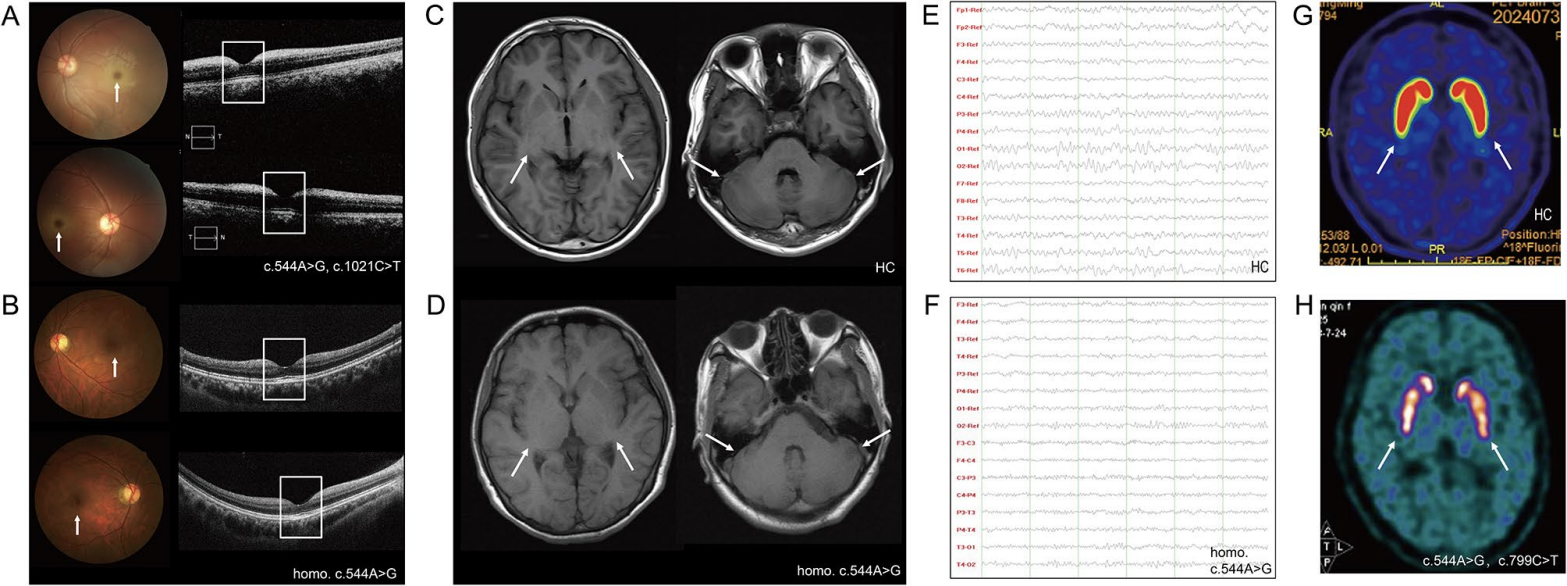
Examination results. (A) Fundus examination showed distinct bilateral cherry-red spots in Patient 2, who had the c.544 A > G, c.1021 C > T genotype. The white arrow indicated the CRS. Optical coherence tomography revealed bilateral diffuse weakness of the retinal nerve fiber layer and ganglion cell complex, and increased reflectivity of the macular inner layer (white rectangular box). (B) No typical cherry-red spots were observed in Patient 8 with the homozygous c.544 A > G genotype. (C) The axial T1 brain magnetic resonance imaging (MRI) of the healthy control was normal. (D) The axial T1 brain MRI of the patient with the homozygous c.544 A > G genotype was normal. The white arrow indicated basal ganglion and cerebellum. (E) The electroencephalogram (EEG) of the healthy control was normal. (F) The EEG of the patient with the homozygous c.544 A > G genotype showed no obvious abnormalities. (G) The DAT-FDG PET result of the healthy control was normal. (H) The DAT-FDG PET result suggested that the distributions of dopamine transporters in the bilateral caudate nucleus and putamen were not obviously abnormal in Patient 6. The white arrow indicated the basal ganglion. *HC* healthy control
